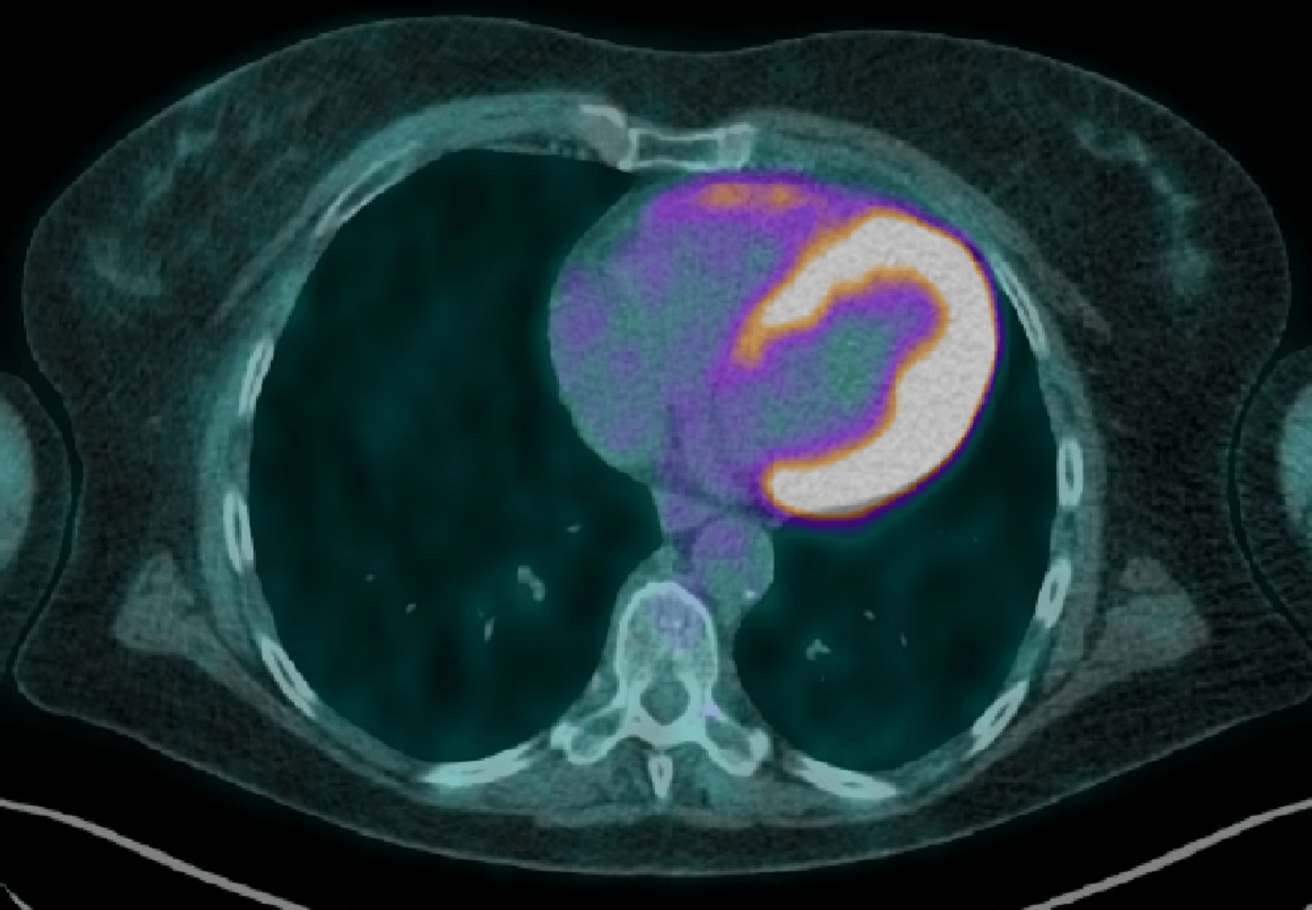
18F-FDG PET-CT after treatment with no hypermetabolic activity, resulting in a complete response 18F-FDG PET-CT: 18F-fluorodeoxyglucose positron emission tomography-computed tomography
